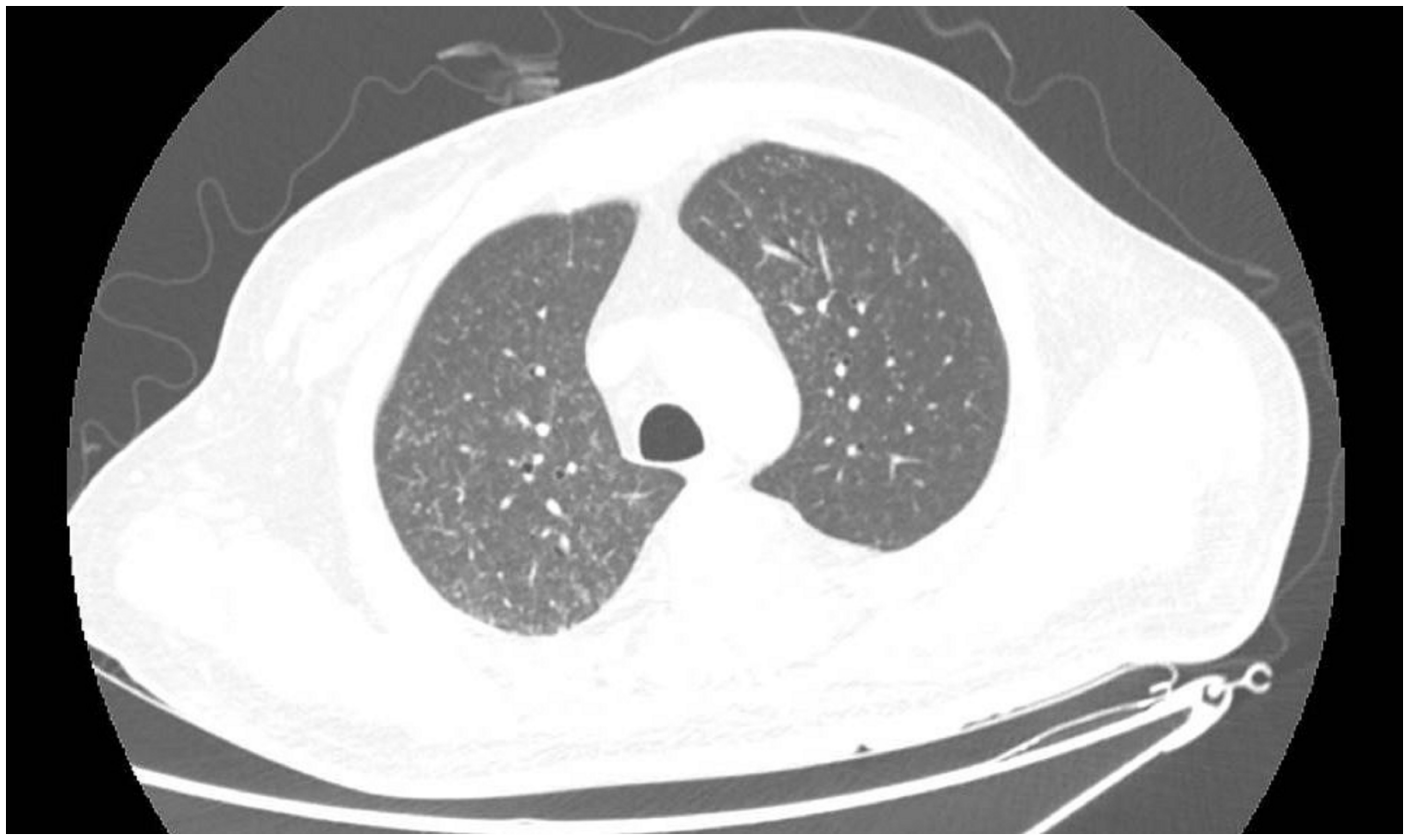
Chest CT scan on admission indicated diffused miliary nodules in bilateral lungs.
